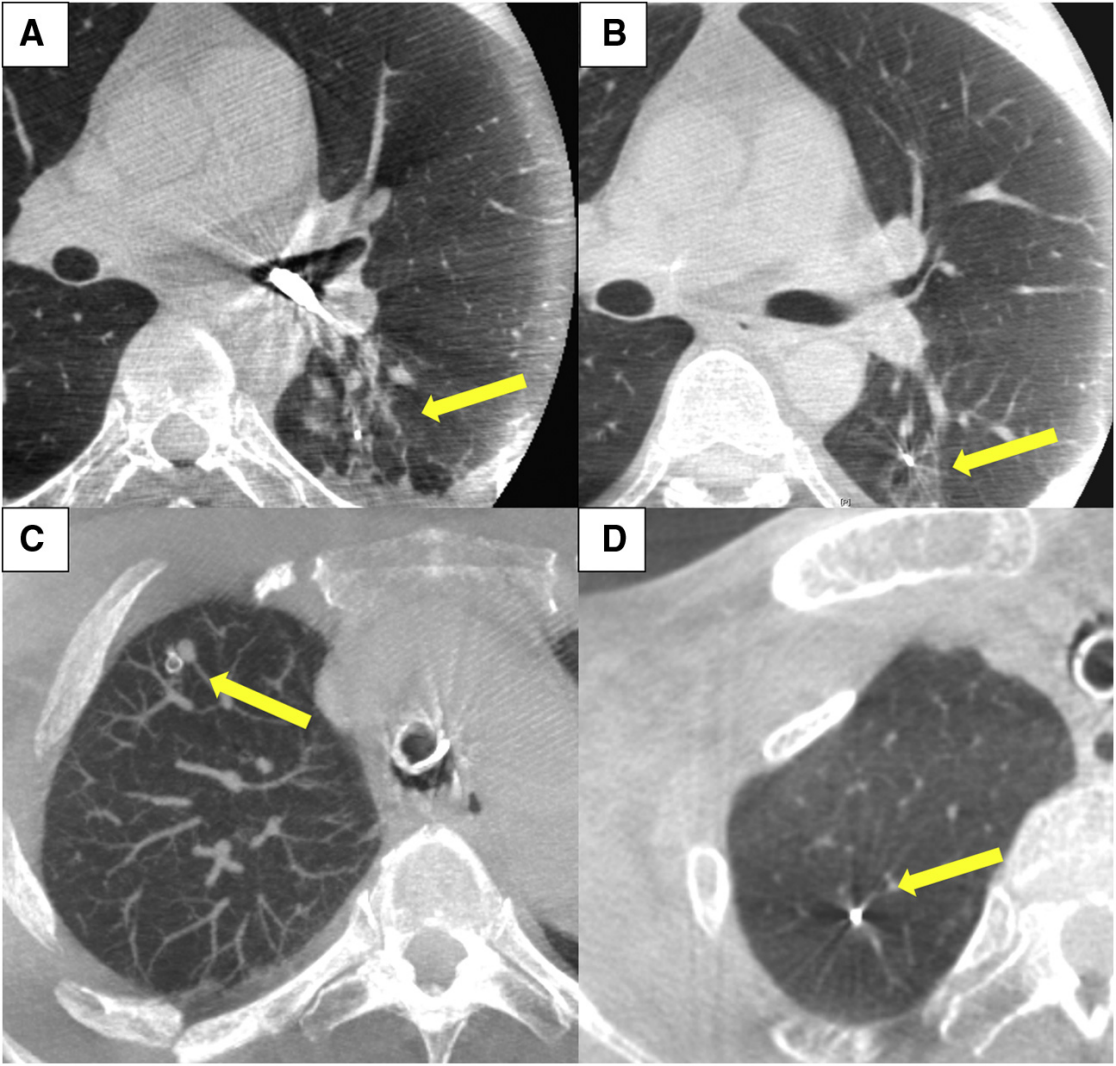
Representative computed tomography (CT) findings of the radiofrequency identification (RFID) marking procedure. A, CT findings of possible intrapulmonary injury associated with device manipulation before releasing the RFID marker. B, CT after marking revealed that the intrapulmonary shadow was less obvious, and there was no apparent intrapulmonary hemorrhage. C, CT after RFID marking revealed that the marker was fixed by a nitinol (NiTi) coil anchor. D, There was no apparent intrapulmonary injury after RFID marking.
